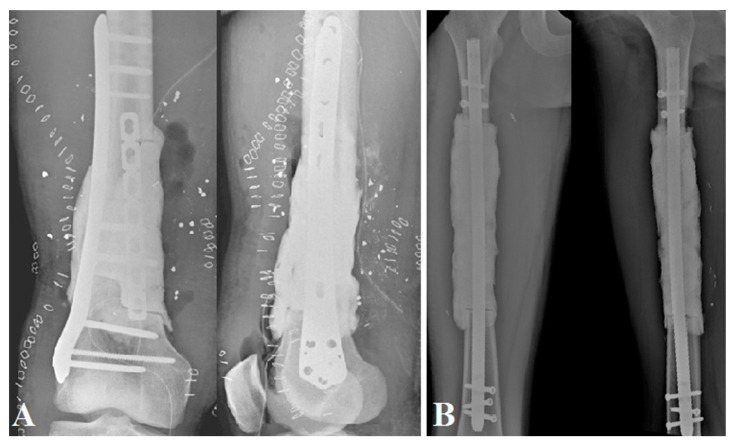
Postoperative X-ray AP and lateral view of the spacers: (A) a plate-enhanced spacer on a bone defect with step-cut in setting a septic ballistic trauma of the right distal femur (patient No. 4); (B) an intramedullary nail-enhanced spacer on a bone defect after an Ewing sarcoma resection.
